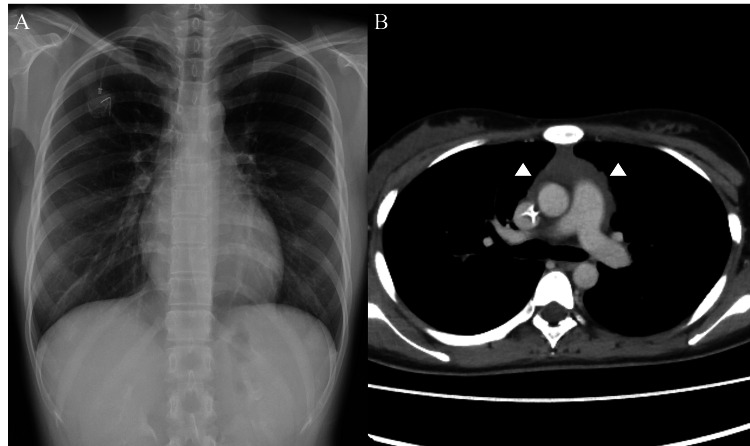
Imaging findings after the completion of four cycles of DA-EPOCH-R A. Chest radiograph after the completion of four cycles of DA-EPOCH-R. B. Contrast-enhanced CT after the completion of four cycles of DA-EPOCH-R The anterior mediastinal mass regressed significantly and was scarcely recognizable on chest radiography CT: computed tomography: DA-EPOCH-R: dose-adjusted etoposide, prednisone, vincristine, cyclophosphamide, doxorubicin, and rituximab
